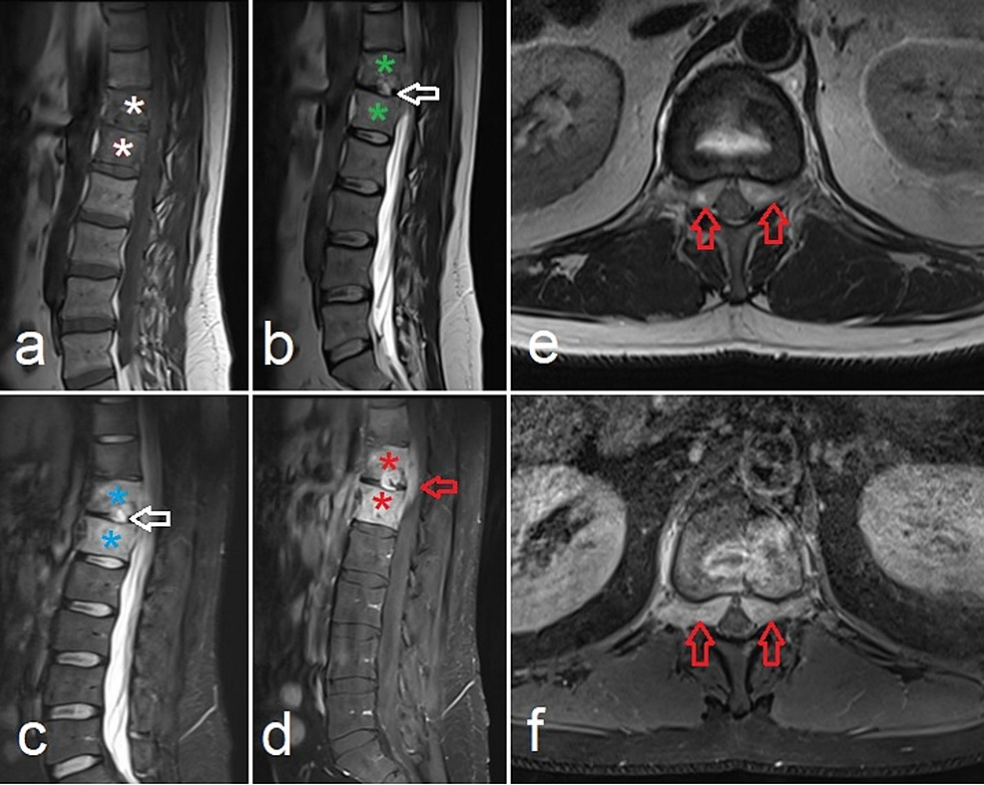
MRI of the lumbar spine shows T1WI hypointense (white asterisks in a), T2WI intermediate (green asterisks in b), STIR hyperintense (blue asterisks in c) signals involving T12-L1 vertebral bodies. Hyperintense signal is also seen in the intervertebral disc protruding into the inferior endplate of the T12 vertebral body, suggesting discitis (white arrow in b and c). T1WI post-contrast sagittal (d) and axial (f) sections show heterogeneous post-contrast enhancement of T12 and L1 vertebrae (red asterisks in d). T2WI intermediate (e): enhancing extraosseous soft tissue mass (d and f) within the spinal canal extending into bilateral lateral recesses and neural foramina surrounding the bilateral traversing L1 and exiting T12 nerve roots (red arrows). T1WI, T1-weighted image; T2WI, T2-weighted image; STIR, short tau inversion recovery
